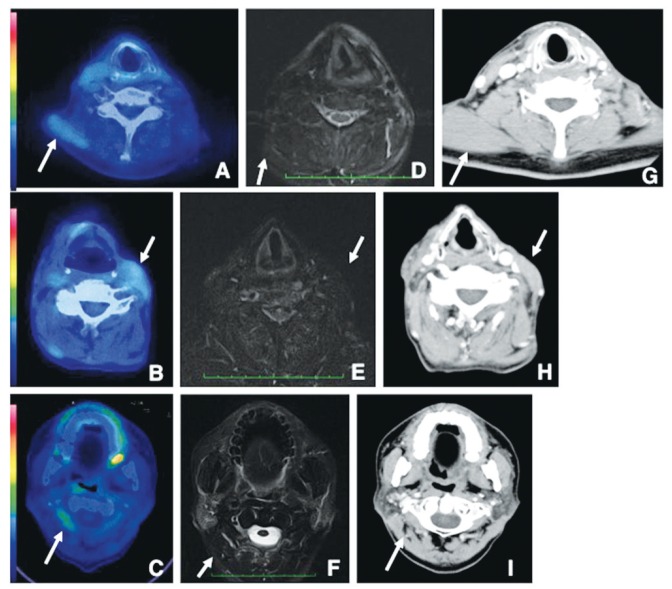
18F-FDG-PET-CT, CT, and MRI examinations of a 51-year-old man 18 months after surgery with ND for SCC of the left side of the mandibular gingiva (A, D, G); of a 78-year-old man 9 months after surgery with ND for SCC on the right side of the tongue (B, E, H); and of a 61-year-old man 35 months after surgery with ND for SCC on the right side of the tongue (C, F, I). 18F-FDG-PET-CT demonstrates increased uptake in the trapezius (SUVmax 2.1) (arrows) (A), sternocleidomastoid (SUVmax 1.9) (arrows) (B), and posterior neck muscles (SUVmax 2.2) (arrows) (C). STIR shows no signal changes of the trapezius (arrows) (D), sternocleidomastoid (arrows) (E), and posterior neck muscles (arrows) (F) in 18F-FDG-PET-positive areas. CT with a soft tissue window shows no density changes of the trapezius (arrows) (G), sternocleidomastoid (arrows) (H), and posterior neck muscles (arrows) (I) in 18F-FDG-PET-positive areas.
